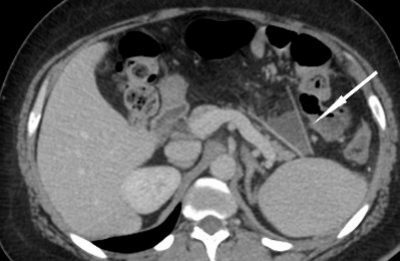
Axial CT post-i.v. contrast 1 week post laparascopic Roux-en-Y gastric bypass using bioabsorbable prosthesis with ﬁbrin glue ﬁxation to prevent a Petersen’s space hernia. Funnel shaped structure (arrow) in Peterson’s defect corresponds to the Gore BioA tissue reinforcement mesh
